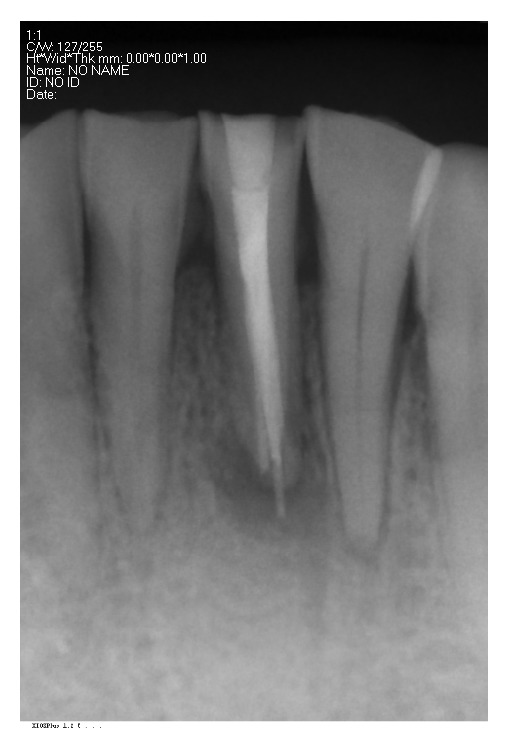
The digital periapical film of the left mandibular central incisor.
